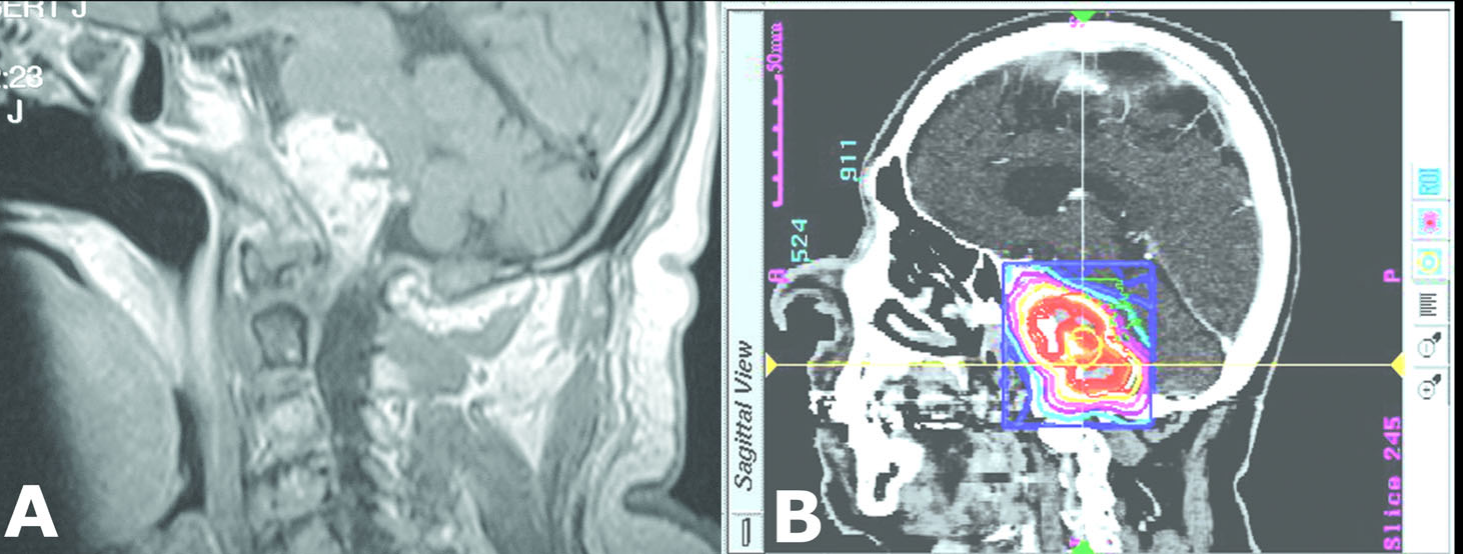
72 year-old man with a history of transitional cell carcinoma with a biopsy proven metastasis to the clivus and foramen magnum. He underwent prior radiation treatment with 60 Gy in 30 fractions. He presented to our institution with progressive facial numbness and difficulty swallowing. (A) Sagittal MRI of the brain after gadolinium administration demonstrating a large clival-based lesion compressing the pons and medulla. Having seen three other skull-base surgeons, none of whom offered surgical resection, we deemed the patient a good radiosurgery candidate. (B) Sagittal CT with treatment contour. The lesion was treated with 2000 cGy in 5 stages. He was followed for 41 weeks when he died of failure to thrive. There was no radiographic progression of this lesion at the time of his last follow-up appointment.
